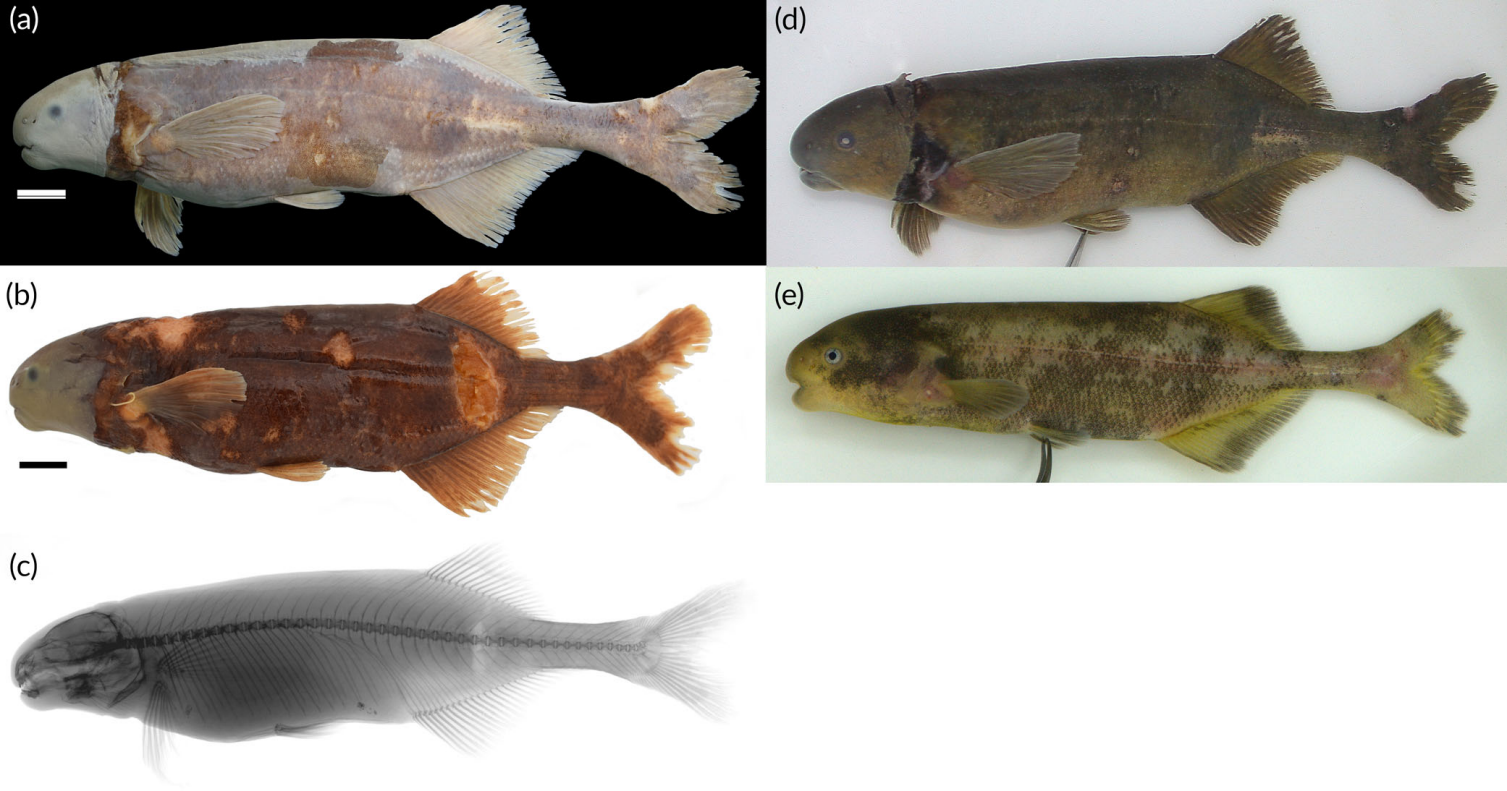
(a) Holotype of *Heteromormyrus ndauorum* sp. nov. (SAIAB 67420), 149.0 mm standard length (SL), from the Mudzira River, Buzi River system, Zimbabwe and Mozambique; (b) paratype of *H. ndauorum* sp. nov. (SAIAB 67420), 135.0 mm SL, from the Mudzira River, Buzi River system, Zimbabwe and Mozambique; (c) X‐ray radiograph of holotype; (d, e) live pictures of *H. ndauorum* sp. nov. Scale bar =1 cm.
